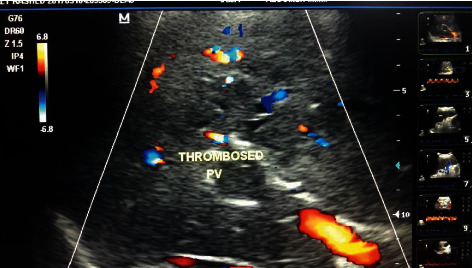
Doppler ultrasound examination of PVT.
